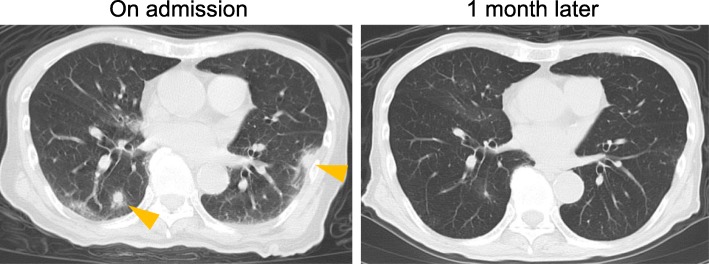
CT scans of septic pulmonary emboli. Chest CT images on admission (left panel). The arrowheads indicate septic embolic lesions. Subpleural ground glass opacity lesions were also present. Pulmonary lesions had resolved in a follow-up CT image at 1 month after the admission (right panel)
